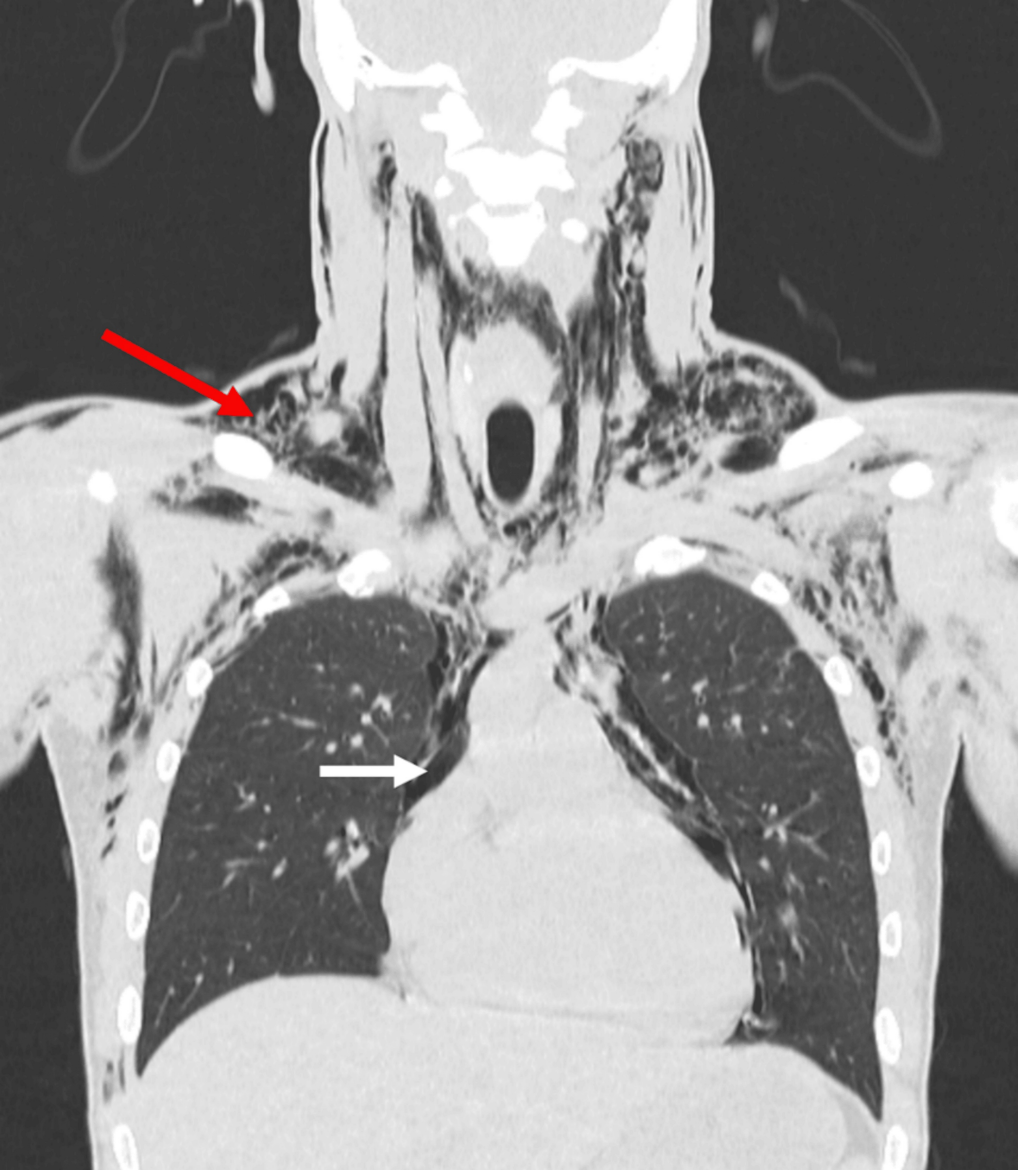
Coronal CT scan showing extensive subcutaneous emphysema in the neck and chest (red arrow) and significant pneumomediastinum (white arrow). CT: computed tomography.
